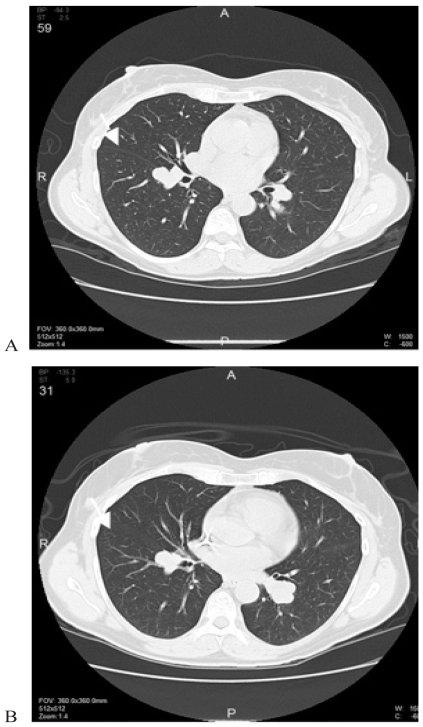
Computed tomography imaging demonstrates no appreciable change in an index lesion (arrow) in the left lung (A) at the time of initiation of therapy with bevacizumab and imatinib, and (B) 6 months after initiation of therapy.
